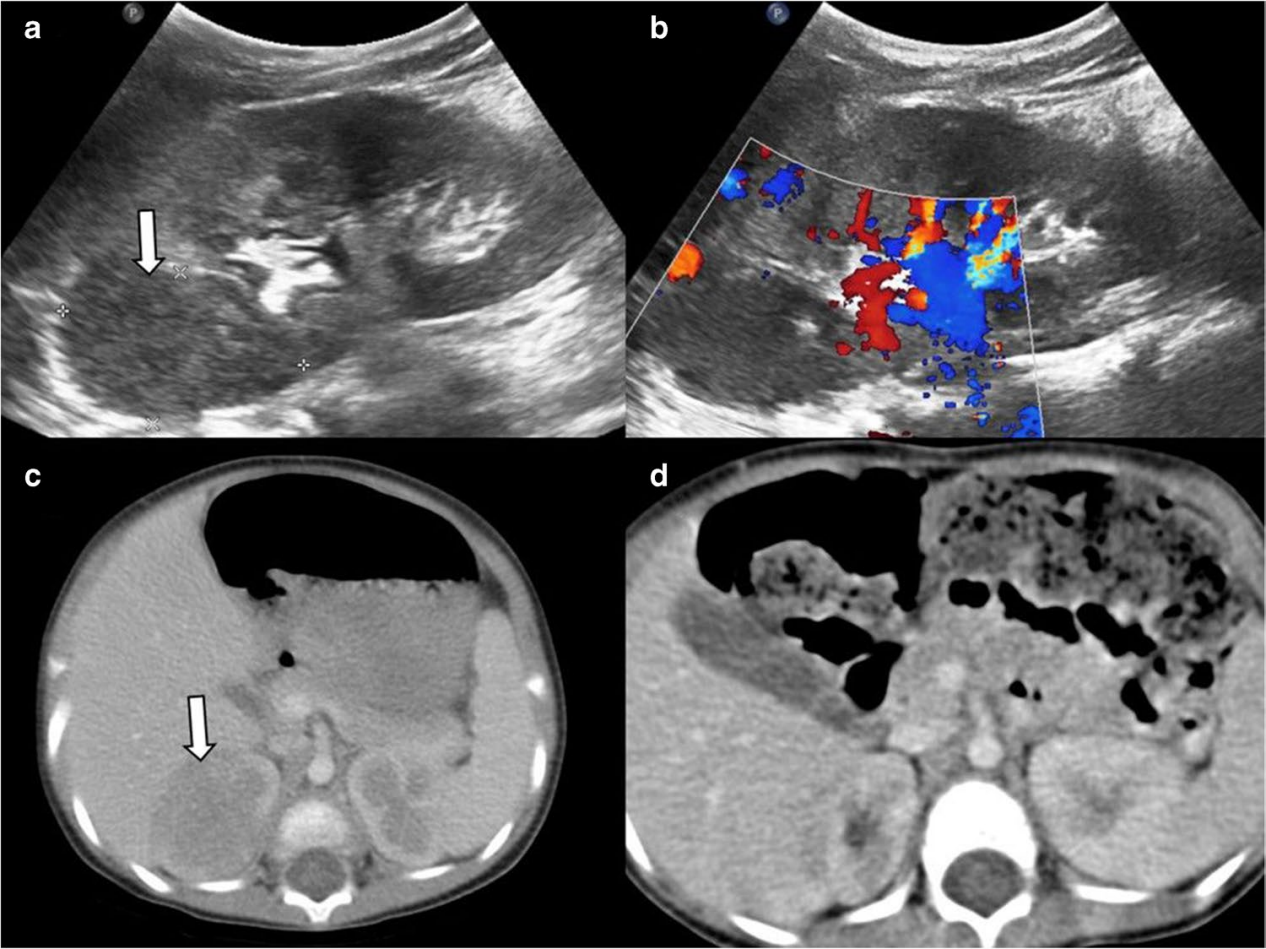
Acute focal bacterial nephritis. Two girls aged 6 years (a, b) and 7 years (c, d) were admitted to hospital with fever and abdominal pain and they had also leukocytosis. a, b Longitudinal grayscale ultrasound (US) image (a) demonstrates a hypoechoic lesion in the upper pole of the left kidney (*arrow*), with no vascularization on color Doppler US (b). The lesion showed resolution with antibiotic therapy (not shown). c Axial contrast-enhanced computed tomography (CT) image shows an ill-defined hypodense lesion (*arrow*) in the upper pole of the right kidney, mimicking a mass. d Three months later, axial contrast-enhanced CT image performed due to suspicion of ileus shows regression of the previously detected hypodense lesion
